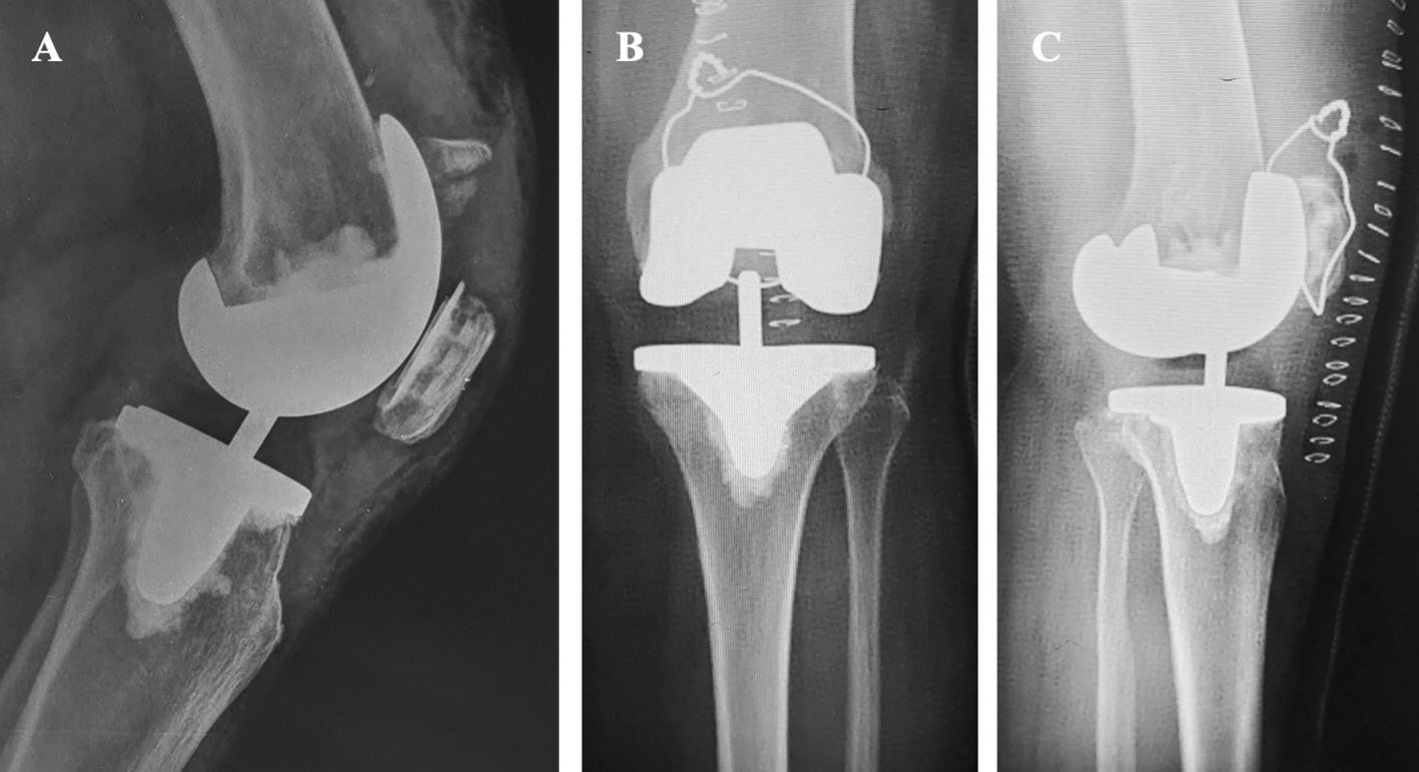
Periprosthetic patellar fracture in the resurfaced setting with failure of the extensor apparatus. A Preoperative lateral X-ray shows a PPPF with extensor mechanism rupture and rotating hinge knee system (Depuy) with stable patellar implant; B Postoperative anteroposterior X-ray view shows the fracture is reduced and fixed with cerclage wiring; C Lateral X-ray view shows good approximation
